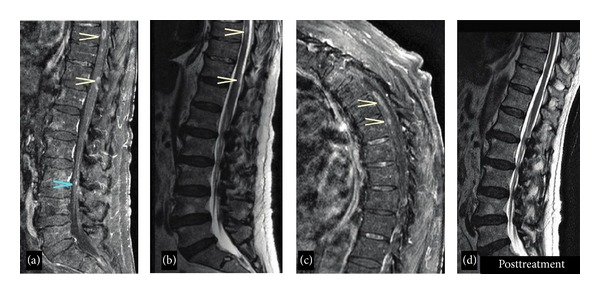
(a) Thoracic and lumbar sagittal MRI and T1 postgadolinium contrast image demonstrating areas of intramedullary enhancement at the levels of T9 and T11, as well as cauda equina enhancement (blue arrow). (b) Sagittal T2 image showing corresponding areas of T2 hyperintensity at the same levels. (c) Sagittal T1 postgadolinium contrast demonstrates enhancement at multiple thoracic levels. (d) Sagittal T2 image after 6 weeks of antiviral treatment demonstrating near resolution of these changes (postcontrast image, not shown, demonstrates resolution of enhancement).
